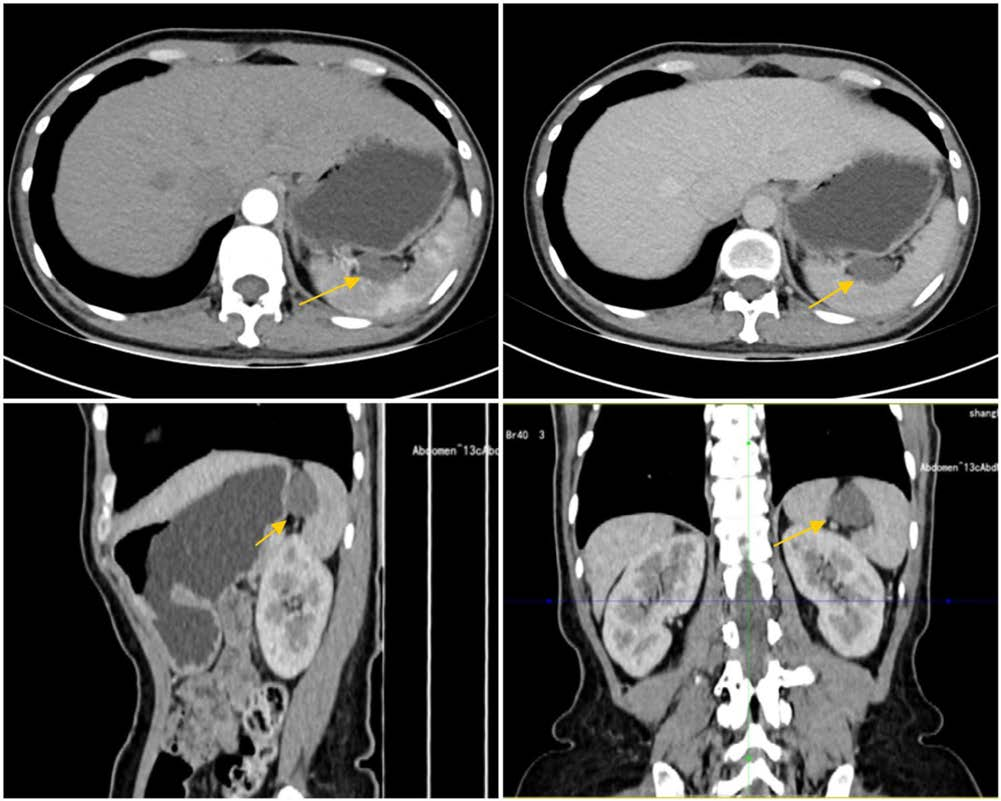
Contrast-enhanced CT images demonstrating the relationship between the tumor and the spleen in axial, sagittal, and coronal planes. CT = computed tomography.
